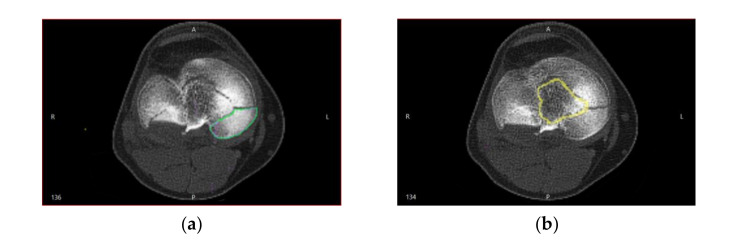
Comminutive tibial fractures shown in CT sections used in the process of the bone fragment fracture surfaces identification. The bone fragment segmented surface is delimited in color: (a) the inside of the bone has a higher intensity than that of the cortex; (b) the estimated surface segmentation is at a distance from the cortical surface.
